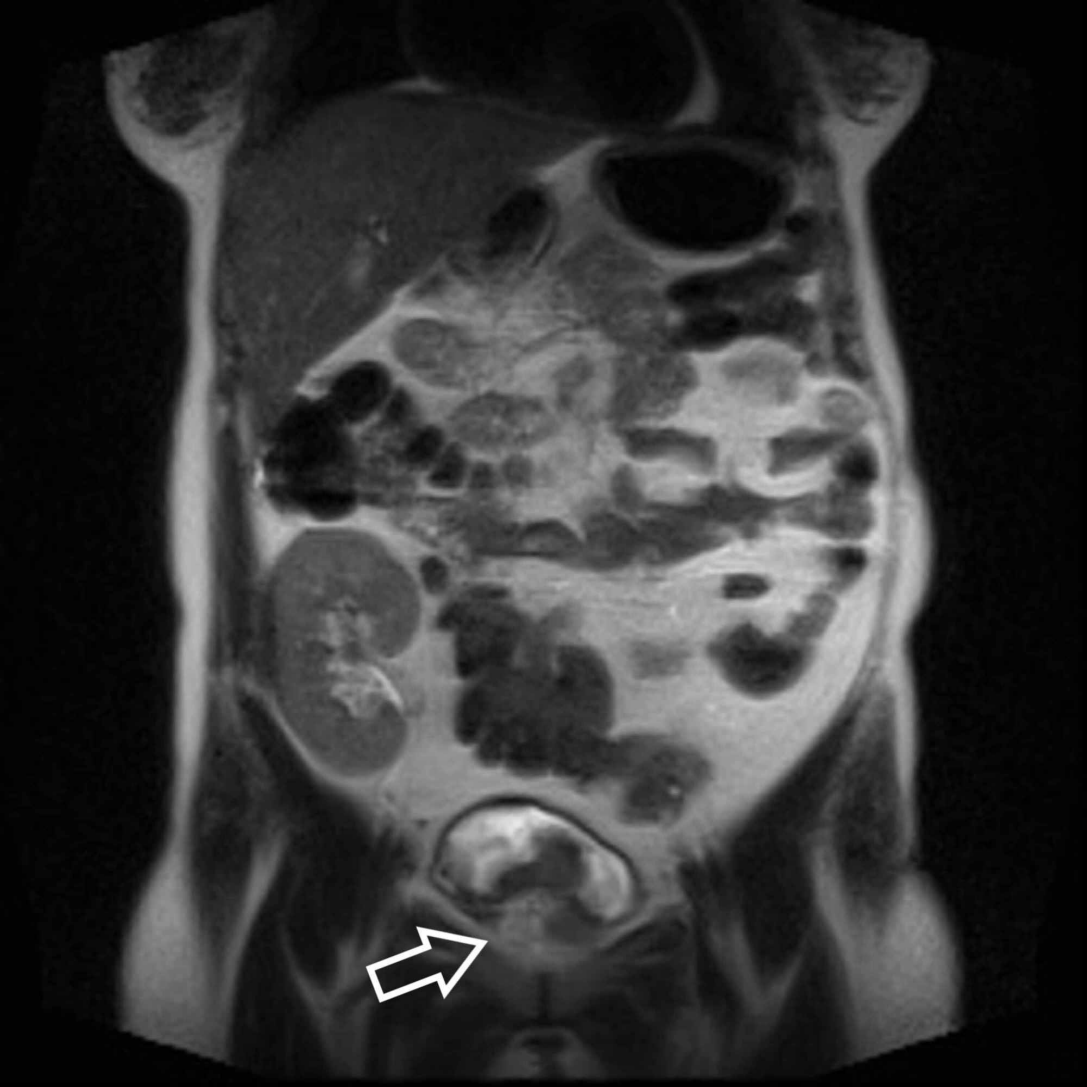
Coronal T2-weighted MRI shows the transplanted kidney in the right iliac fossa and extravesical extension of bladder mass (white arrow). MRI, magnetic resonance imaging
